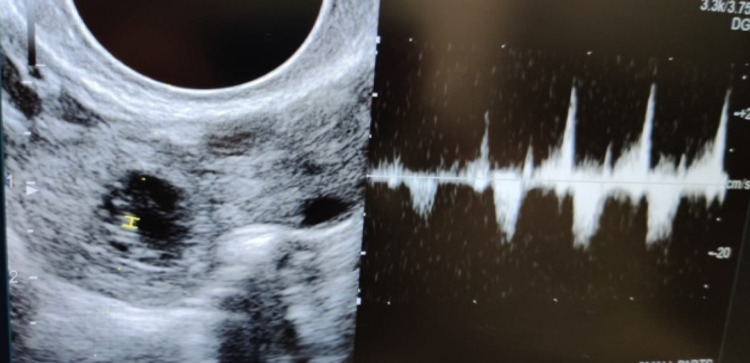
M mode of ultrasonography.
